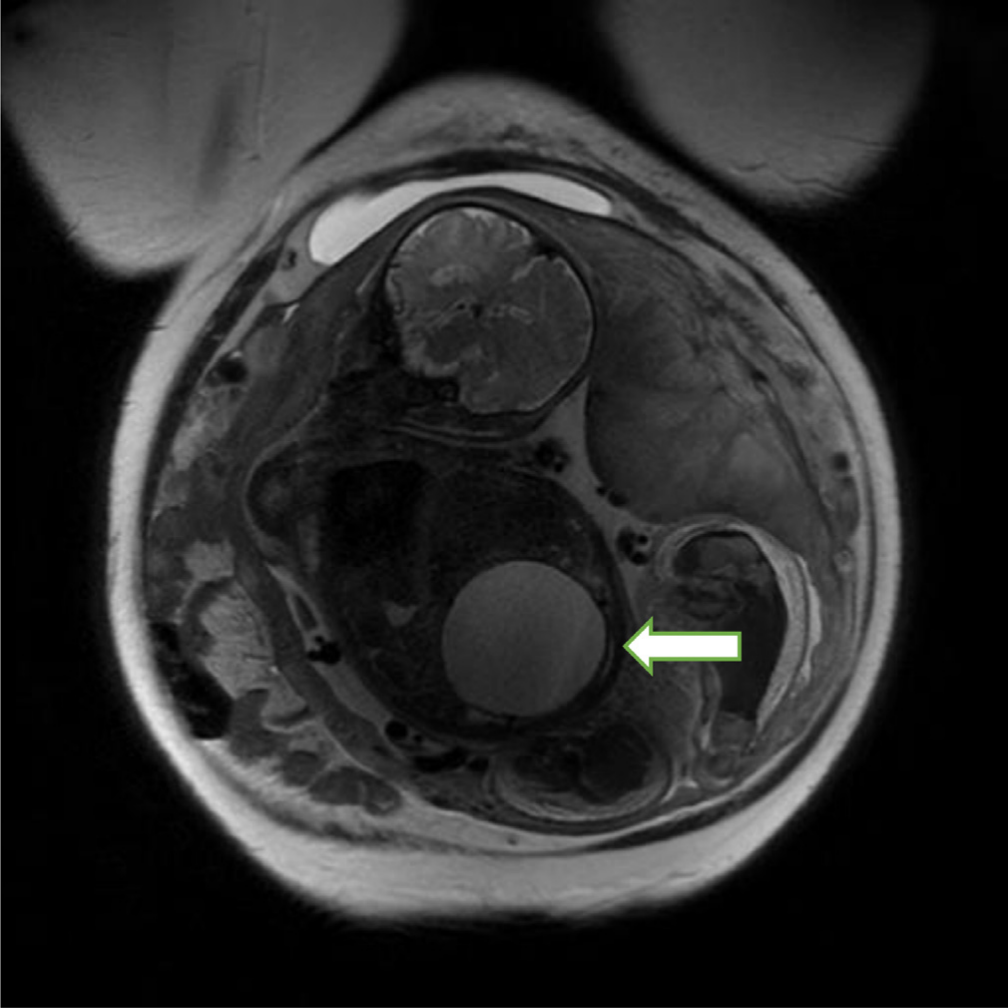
Fetal MRI at 34 weeks showing worsening obstructive uropathy (white arrow).
